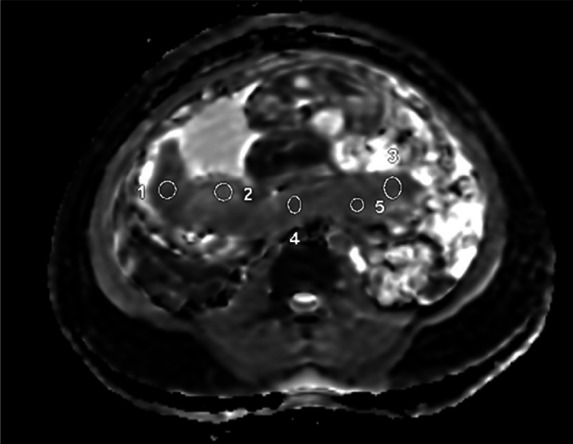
A 25-year-old female at 30 weeks of gestation was referred for placental magnetic resonance imaging (MRI) to evaluate intrauterine growth restriction (IUGR). The MRI revealed an ADC value of 1.734 (10^−3^ mm^2^/s). The ADC map shows the localization of the Region of Interest (ROI) within the placenta
